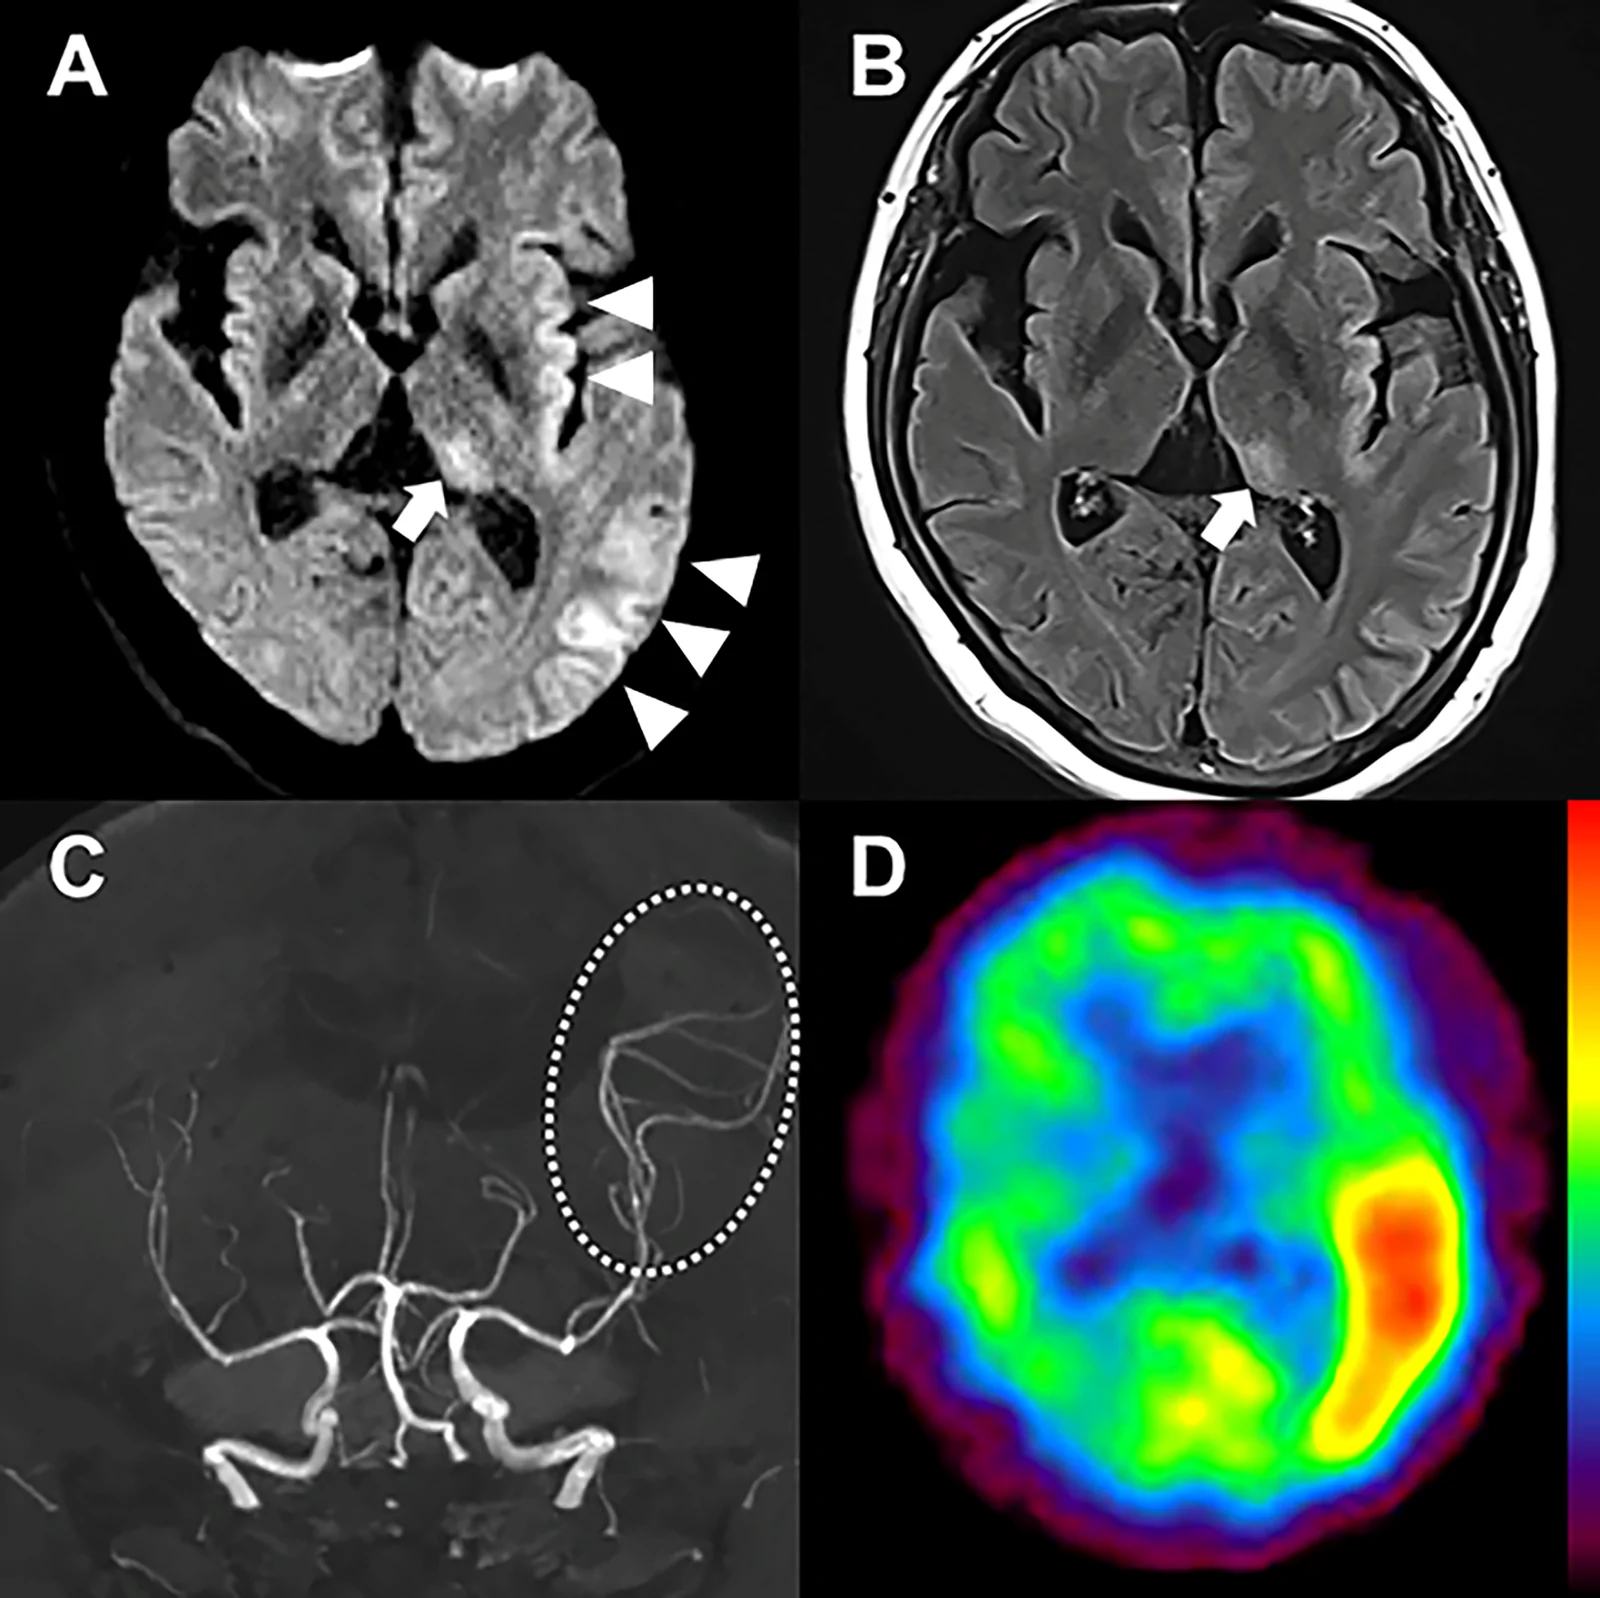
Imaging findings on the first admission. (A) Diffusion-weighted imaging (DWI) shows hyperintensity in the left cerebral cortex (white arrow heads) and left pulvinar of the thalamus (white arrows). (B) Fluid-attenuated inversion recovery imaging presents hyperintensity in the same regions as seen on DWI (white arrow) and hypointensity in the subcortical white matter in the left temporal lobe. (C) Magnetic resonance angiography reveals increased vascularity in the left middle cerebral artery territory (white dotted line). (D) Iodine-123-labeled N-isopropyl-p-iodo-amphetamine single-photon emission computed tomography shows hyperperfusion in the left temporal and parietal lobes.
